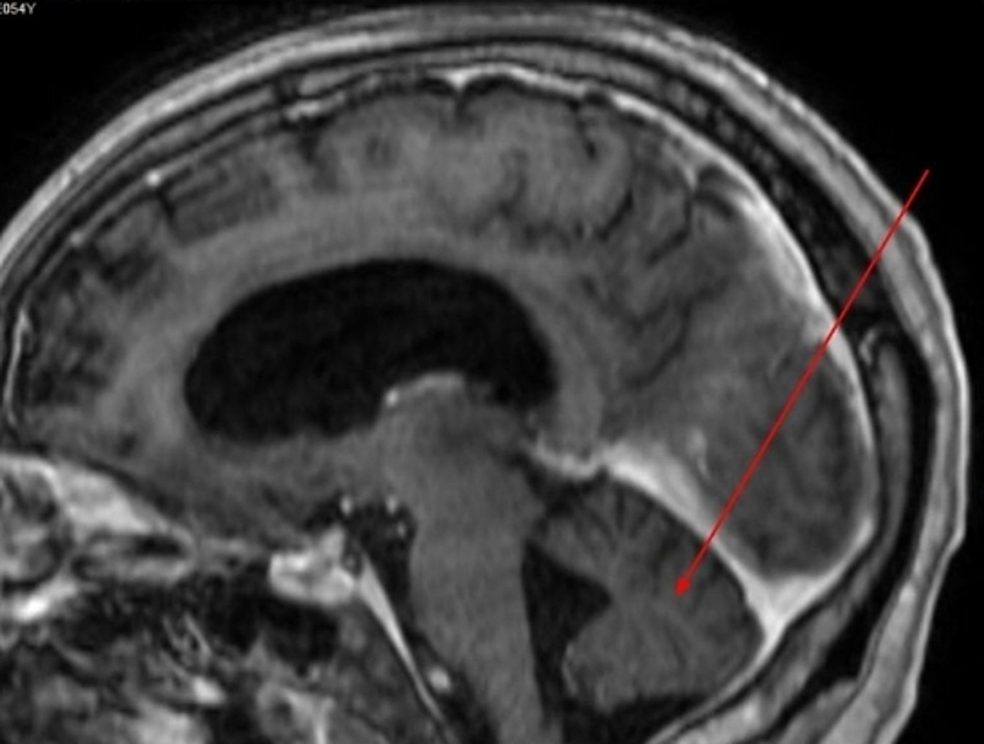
Brain MRI, multiplanar reconstructed sagittal Sagittal brain MRI, showing cerebellar atrophy (red arrow).
